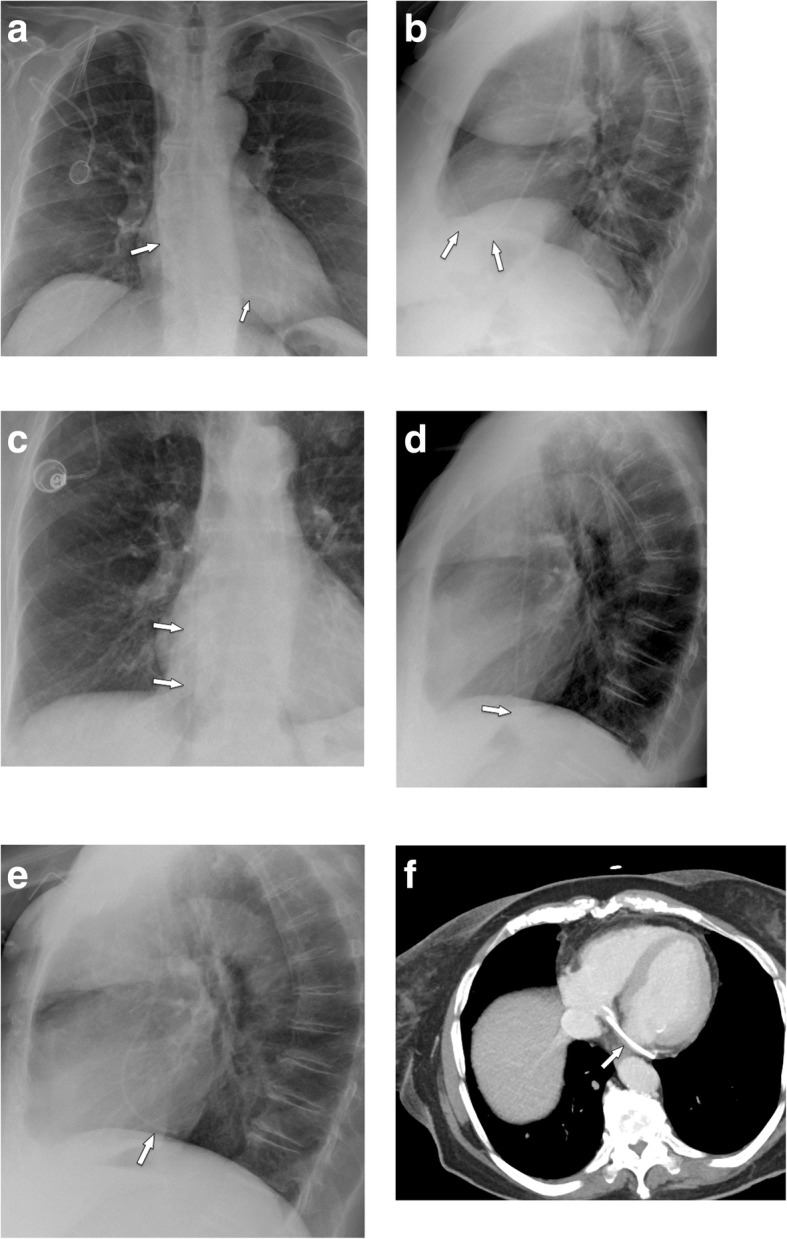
Malpositioning in the heart. a PA chest radiography shows a very long, looping catheter, which had not been shortened by the surgeon during the procedure. The tip is projecting onto the right heart (arrow). b Lateral chest radiograph shows typical anterior bending (arrows) due to position in the right ventricle. c In this example, the catheter tip is not clearly seen, but seems to be placed too deep (arrows). d Lateral projection shows a straight course, with the tip in the inferior vena cava (arrow). e Five weeks later, a follow-up film now shows posterior curving of the catheter. f Contrast-enhanced MDCT (MIP reconstruction) confirms displacement of the catheter into the coronary sinus
